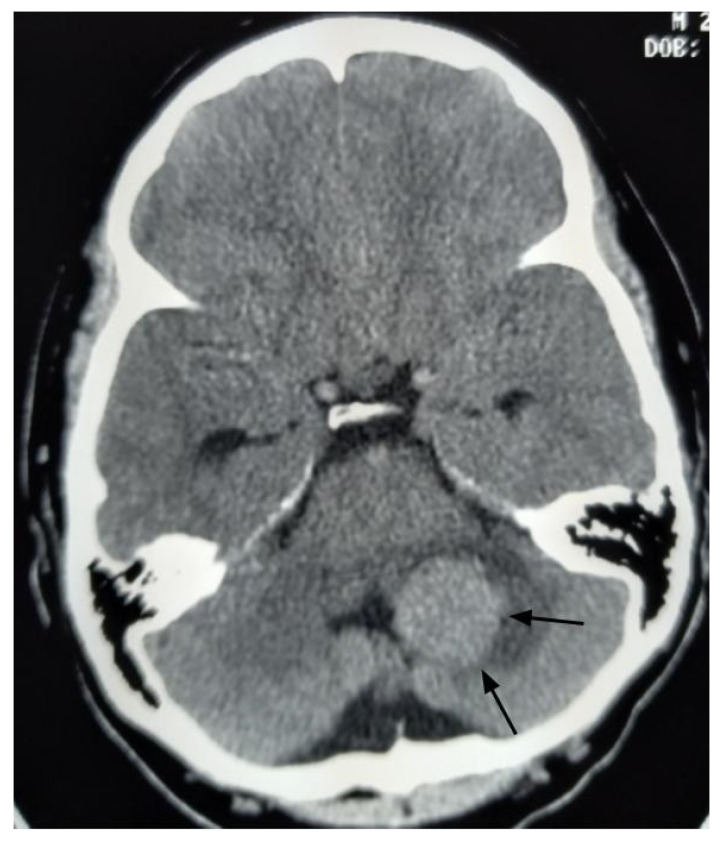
Axial head computed tomography with contrast image, performed before the initial surgery, reveals a large enhancing mass in the left cerebellum (black arrows).
